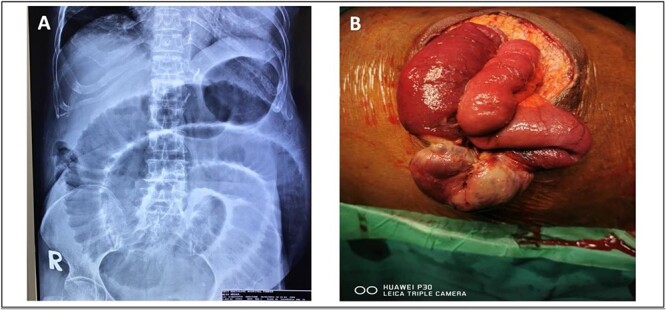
(A) Plain abdominal radiograph showed dilated small bowel (B) intraoperative finding of unhealthy sloughy small bowel segment in the hernia sac and pus was present.
